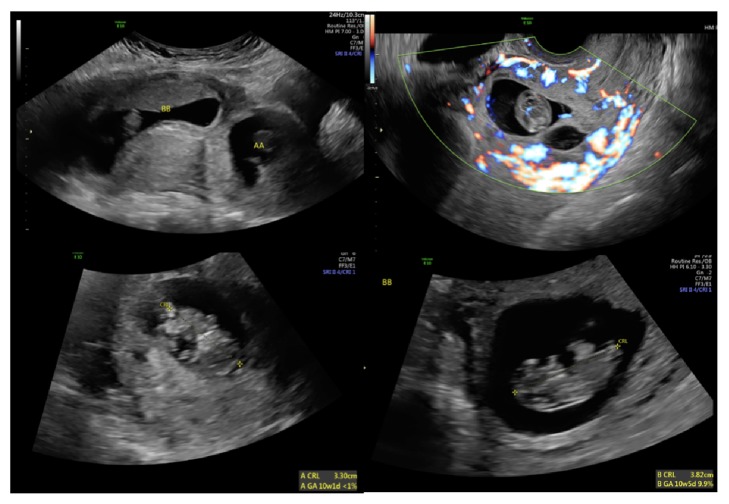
Sonographic imaged before selective reduction of cesarean scar pregnancy with potassium chloride (KCl injection). Upper left demonstrates cesarean scar pregnancy (AA) and intrauterine pregnancy (BB). Upper right demonstrates that cesarean scar pregnancy in close proximity to serosal edge of the uterus. Lower right and left demonstrate live pregnancy of Twin A (cesarean scar pregnancy) and Twin B (intrauterine pregnancy).
